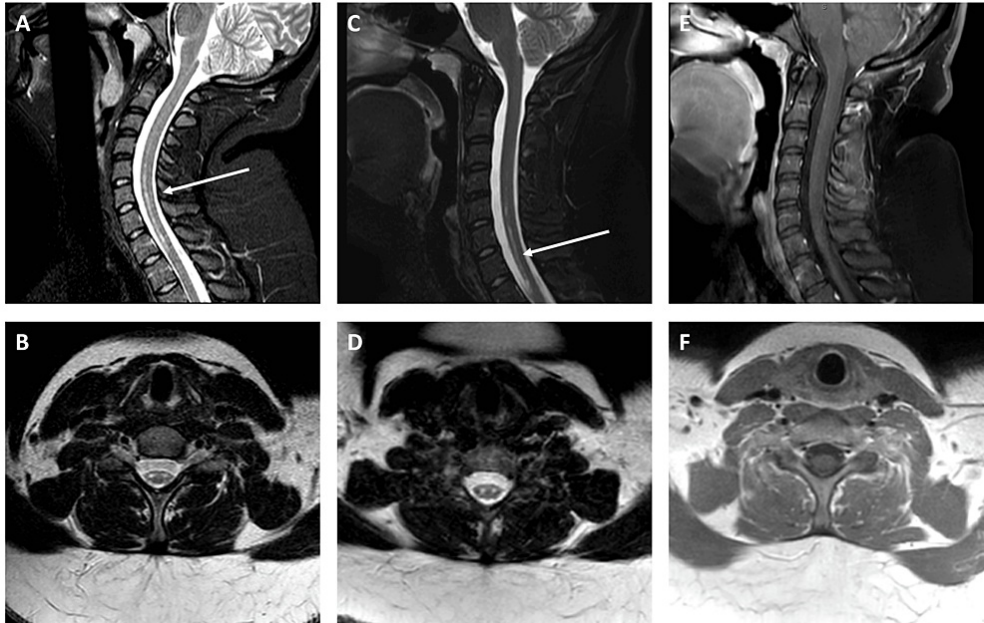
Initial and repeat magnetic resonance imaging (MRI). MRI during the initial hospitalization and repeat imaging. (A) MRI cervical spine (C-spine) sagittal T2 STIR imaging taken at the time of hospitalization shows signal changes from C5 to T1. The white arrow highlights the cord signal changes. (B) MRI C-spine axial T2 turbo spin-echo (TSE) imaging, taken at the time of hospitalization around the C7 vertebral body, reveals signal changes in the anterior columns. (C) MRI C-spine sagittal T2 STIR imaging taken seven months after the episode shows signal changes from C5 to T1. The white arrow highlights the cord signal changes. (D) MRI C-spine axial T2 TSE imaging taken seven months after the episode at approximately C7 vertebral body shows signal changes at the anterior columns. (E) MRI C-spine sagittal T1 post-contrast imaging taken seven months after the episode does not show any contrast enhancement within the spinal cord. (F) MRI C-spine axial T1 post-contrast imaging taken seven months after the episode at approximately C7 vertebral body does not show any contrast enhancement within the spinal cord.
